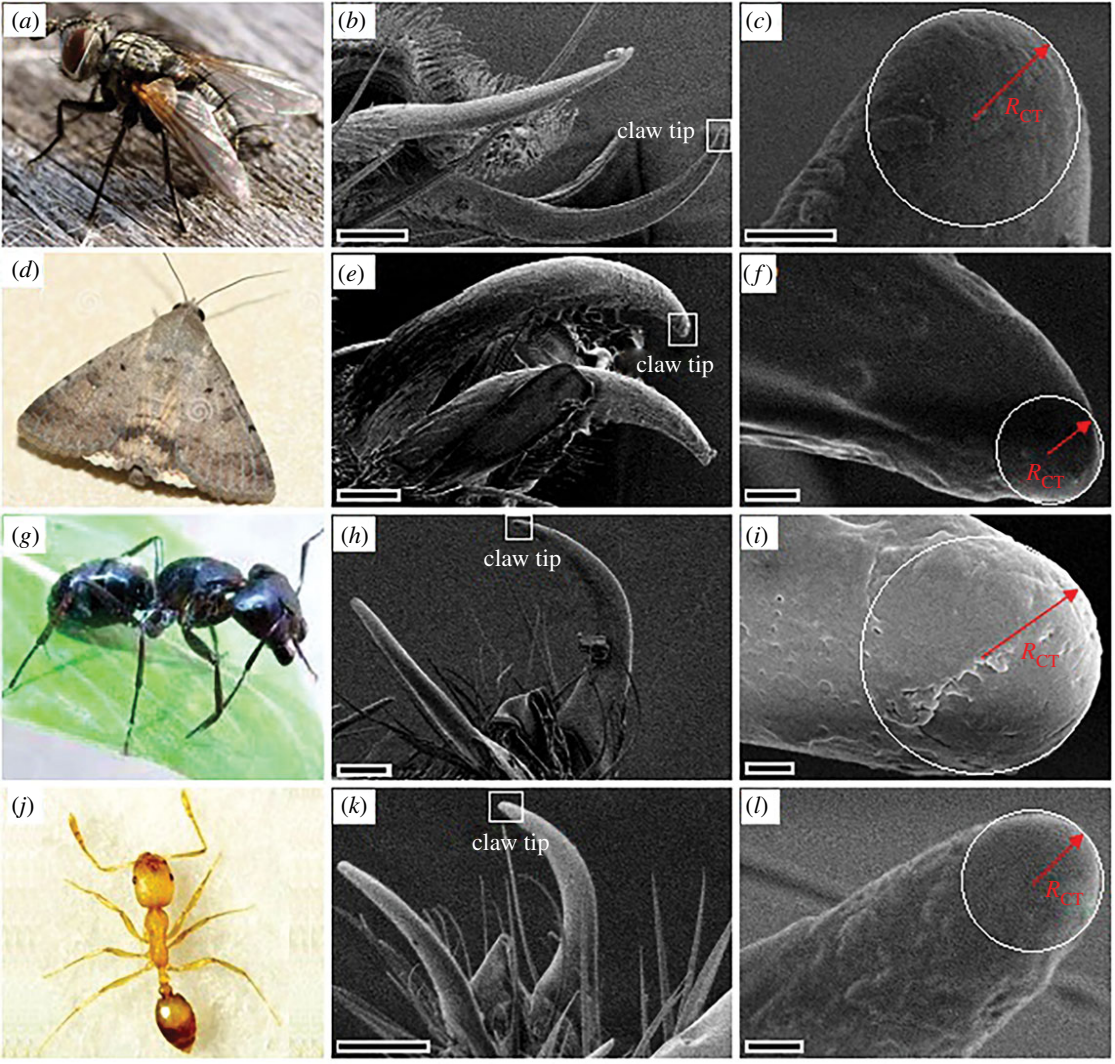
Attachment organs. (*a*,*d*,*g*,*j*) The selected insects. (*b*,*e*,*h*,*k*) General morphology of claws, black bars represent 25 µm. (*c*,*f*,*i*,*l*) Hemisphere-shaped claw tips, black bars represent 1 µm. $R_{\mathrm{CT}}$, claw tip radius. (*a*–*c*) Fly *Mu. domestica*. (*d*–*f*) Moth *Noctuidae insect*. (*g*–*i*) Ant *Cam. japonicus*. (*j*–*l*) Ant *Mo. pharaonis*.
